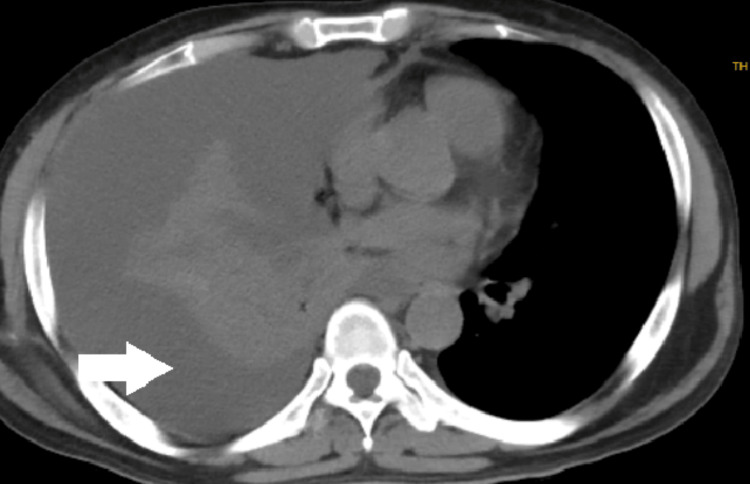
Chest CT-gross right pleural effusion (white arrow) with mild mediastinum shift to left with underlying lung collapse
